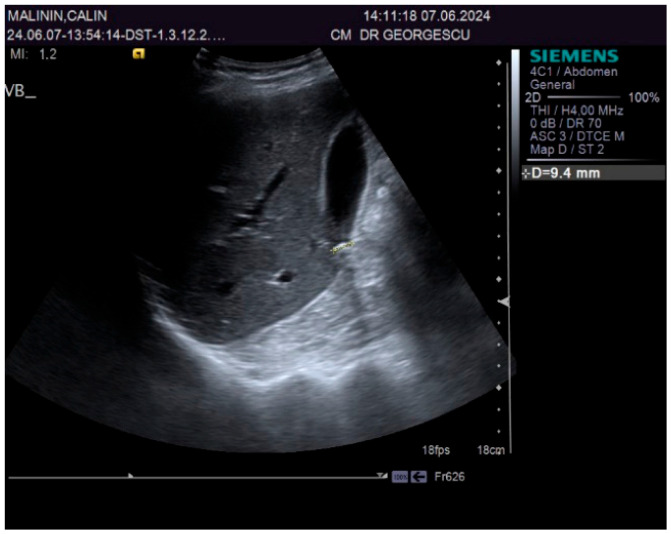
Ultrasound aspect: diffuse liver steatosis.
